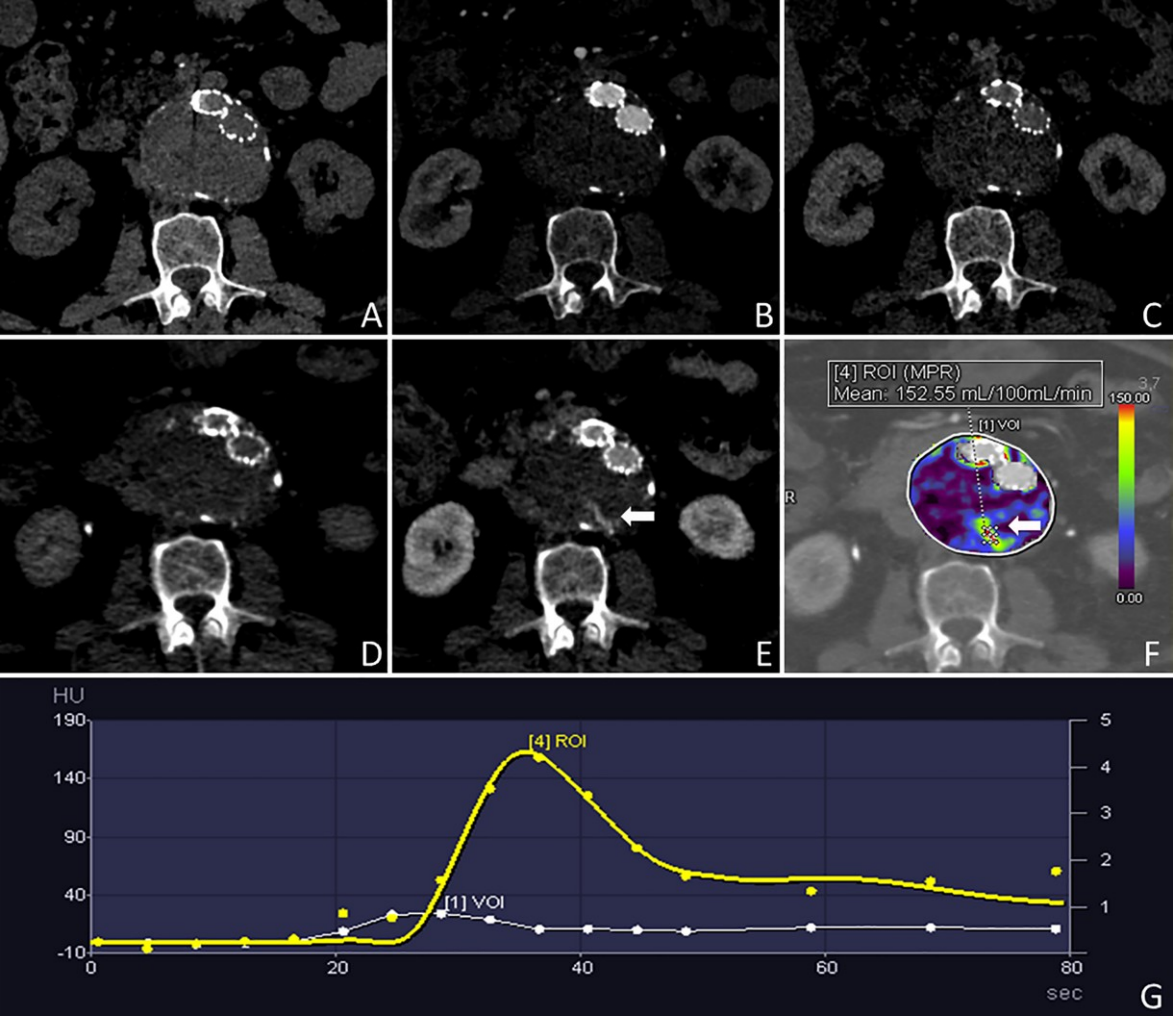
Case demonstration. 76-year-old man with a BMI of 30.85 kg/m^2^ who had undergone EVAR 2.5 years prior to the CTA examination. The non-contrast, arterial and venous static CTA acquisitions (A, B and C, respectively) do not show the presence of any endoleaks. The dCTA images acquired 2 and 38 seconds after the contrast media injection (D and E, respectively) show a type II endoleak, fed by a lumbar artery, that is also depicted by the color-coded perfusion maps (arrow in E and F). The TAC shows the kinetics of the endoleak (G) characterized by a wash-in that starts after 20 seconds, reaching a peak at 36 seconds, and then a rapid wash-out that does not allow its detection with the static CTA acquisitions.
